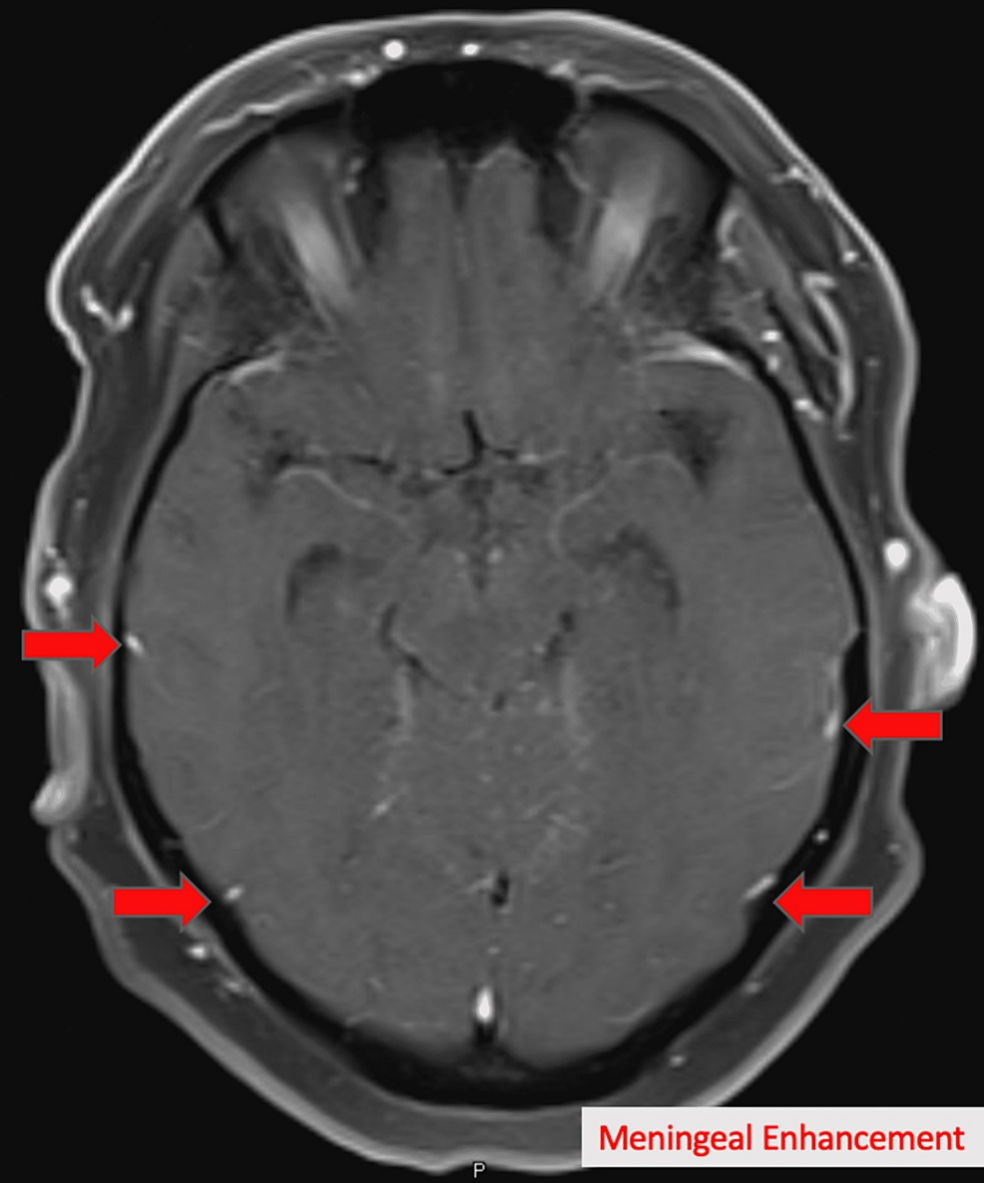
MRI of head showing new onset meningeal enhancement (arrows indicating enhancements).
